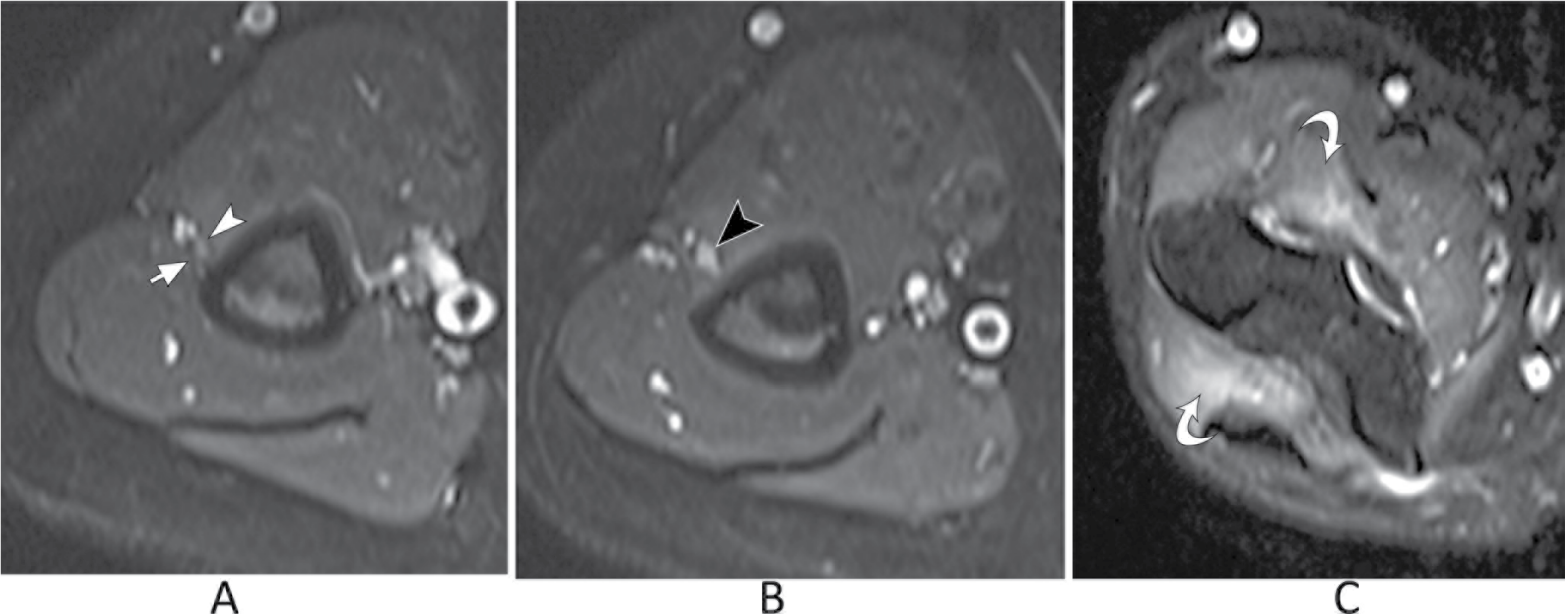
Radial nerve compression at the spiral groove. Axial T2-weighted fat suppressed images at the level of the humeral spiral groove (A and B) and the level of the elbow joint (C). In (A), the ulnar nerve has normal size and signal (white arrowhead) and is starting to cross the lateral intermuscular septum (white arrow). At a slightly more distal image, there is increased signal and thickening of the radial nerve (black arrowhead). There is denervation edema of the brachialis and supinator muscles (curved arrows)
